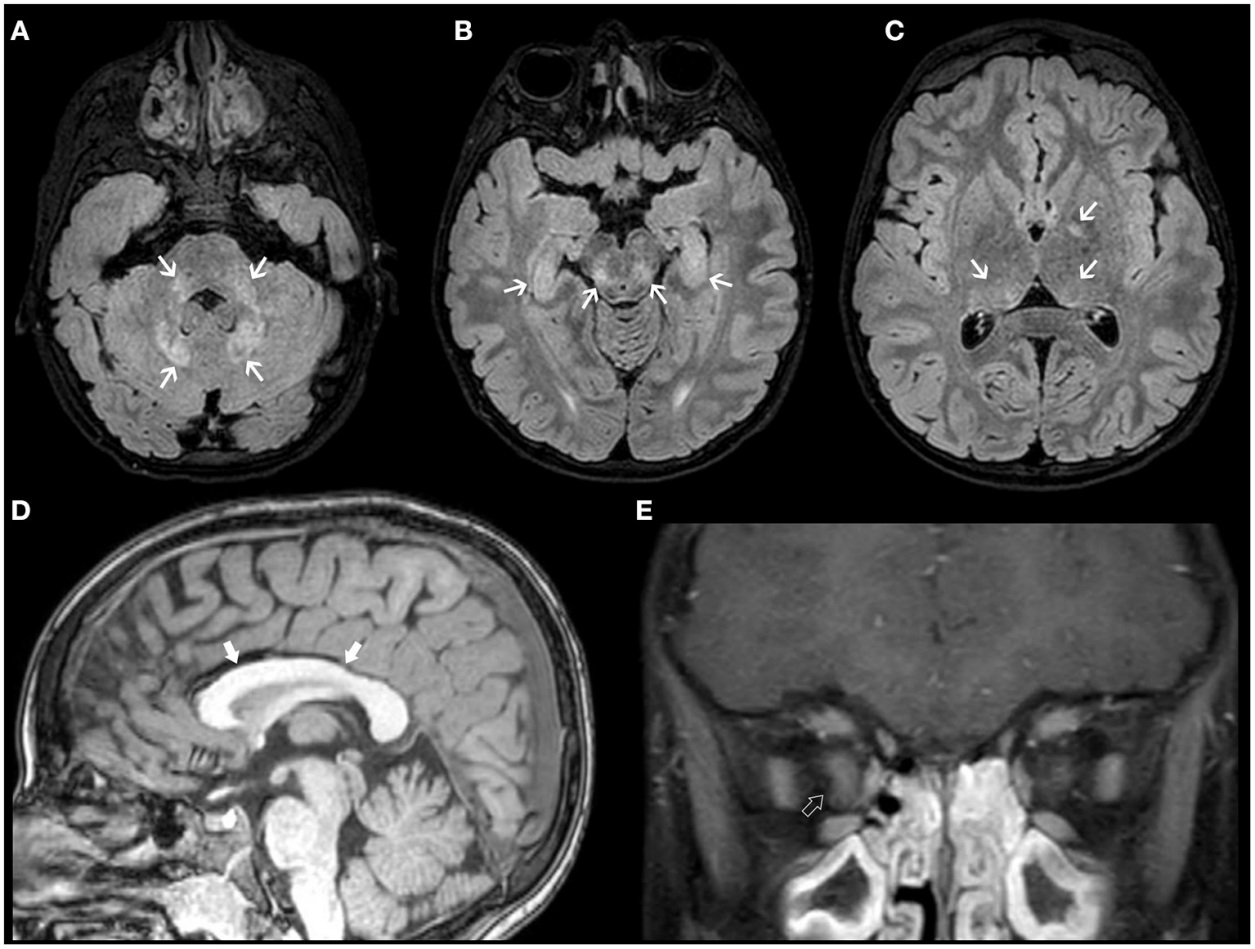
Imaging characteristics of Neurofibromatosis Type 1 (NF1). Axial FLAIR (A–C) and sagittal T1 WI (D) of an 18-year-old boy with NF1 reveals multiple focal areas of abnormal hyperintensity (FASI) (white arrows) distributed in the dentate nuclei, middle cerebellar peduncles, mesencephalic tegment, hippocampi, and pulvinar bilaterally as well as left globus pallidus. There is also diffuse thickening of the corpus callosum (thick white arrows). Coronal contrast-enhanced T1WI FAT SAT of the orbits (E) of the same patient show thickening, tortuosity, and enhancement of the intra-orbital segment of the right optic nerve (open arrow), in keeping with an ipsilateral optic nerve glioma.
